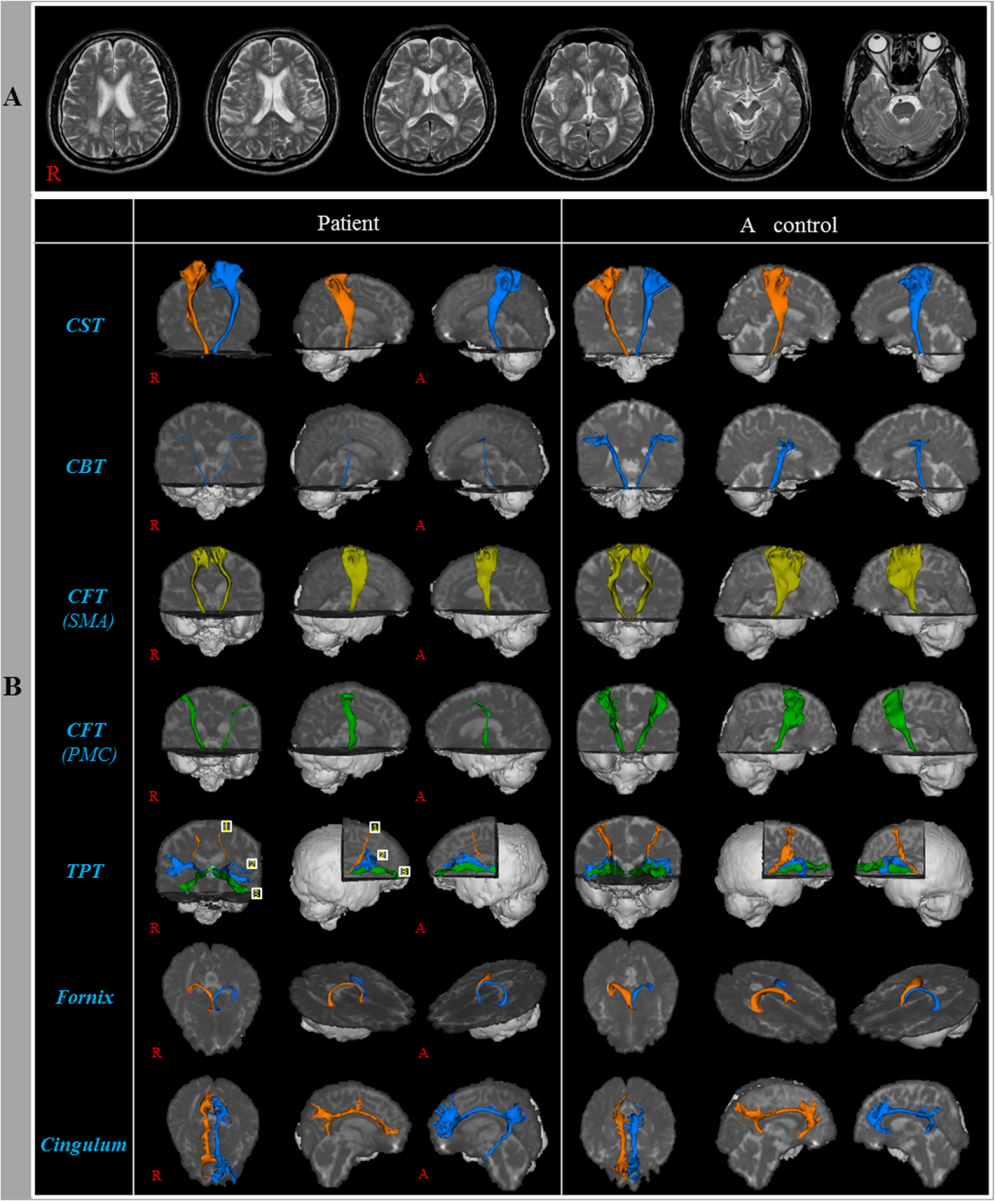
a T2-weighted brain MR images obtained at admission show high signal intensity lesions in the subcortical gray matter and periventricular white matter. b Diffusion tensor tractographies for the patient and a control volunteer man (58-year old male). CST: Corticospinal tract, CBT: Corticobulbar tract, CFT: Corticofugal tract, SMA: Supplementary motor area, PMC: Premotor area, TPT: Thalamoprefrontal tract; 1: Dorsolateral prefrontal cortex, 2: Ventrolateral prefrontal cortex, 3: Orbito prefrontal cortex
